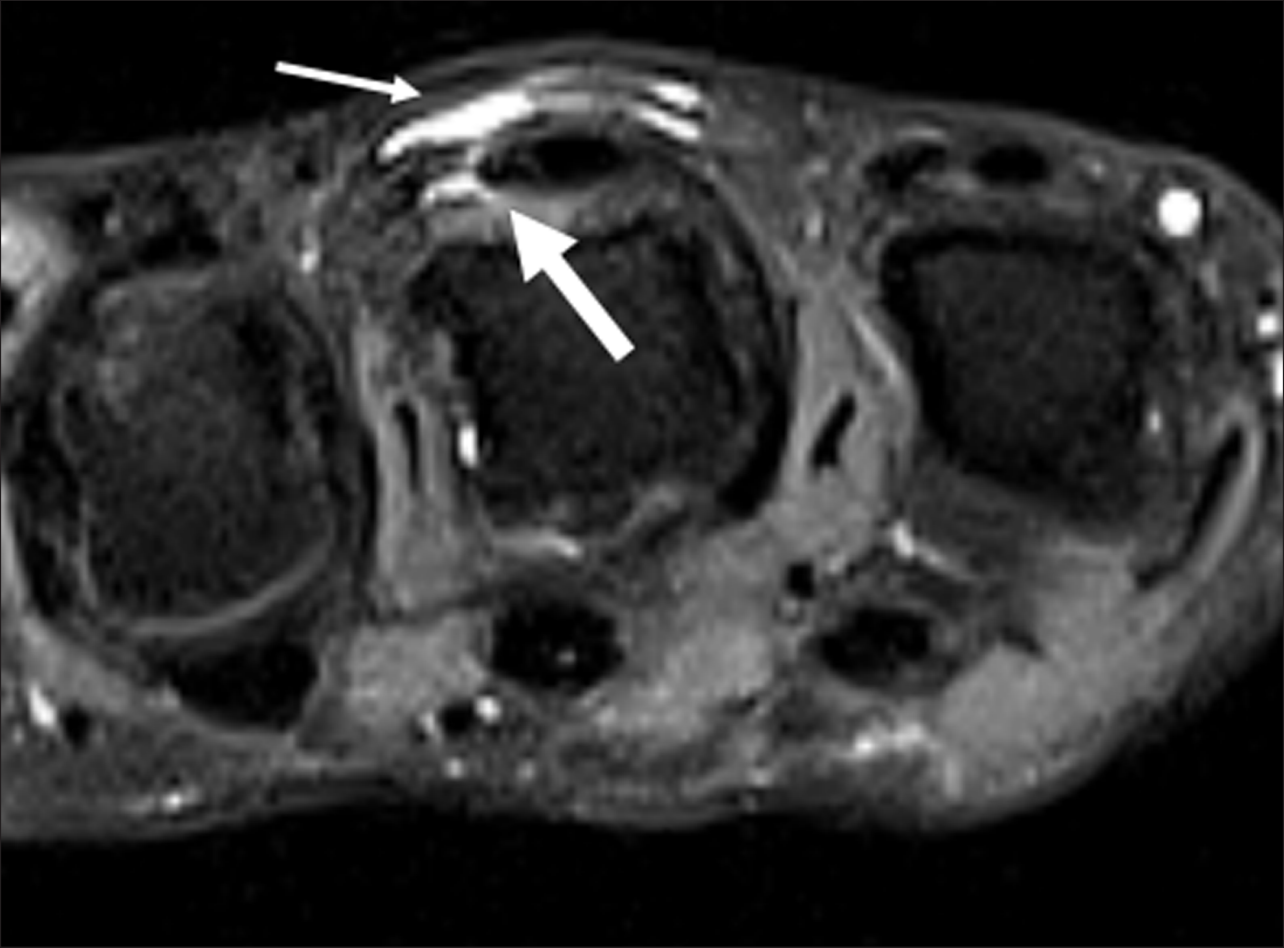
Subsequent dynamic ultrasound was performed. This examination confirms the focal disruption of the ulnar dorsal cap of the extensor tendon (*Figure 2*, bold arrow) leading to radial subluxation with contiguous fluid collection on the dorsal side at the level of the head of the third metacarpal (*Figure 3*, thin arrow).
